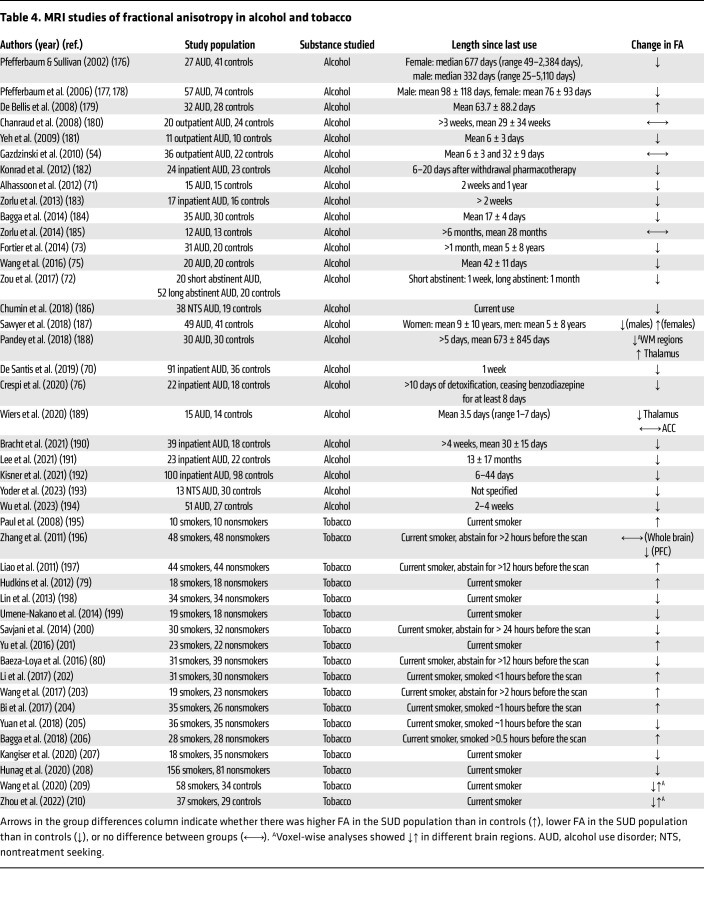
MRI studies of fractional anisotropy in alcohol and tobacco
